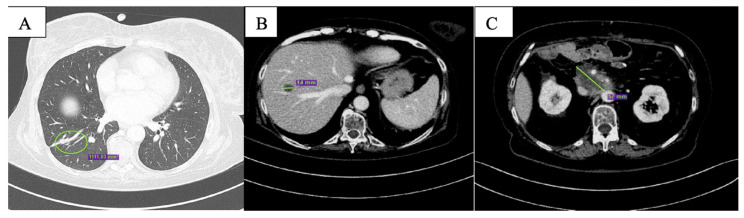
CT scan after the first 2 months of olaparib showing complete response of the nodular lesion in inferior lobe of the right lung (A), reduction in size of the hepatic lesion in the 8th segment (B) and of peripancreatic tissue (C).
